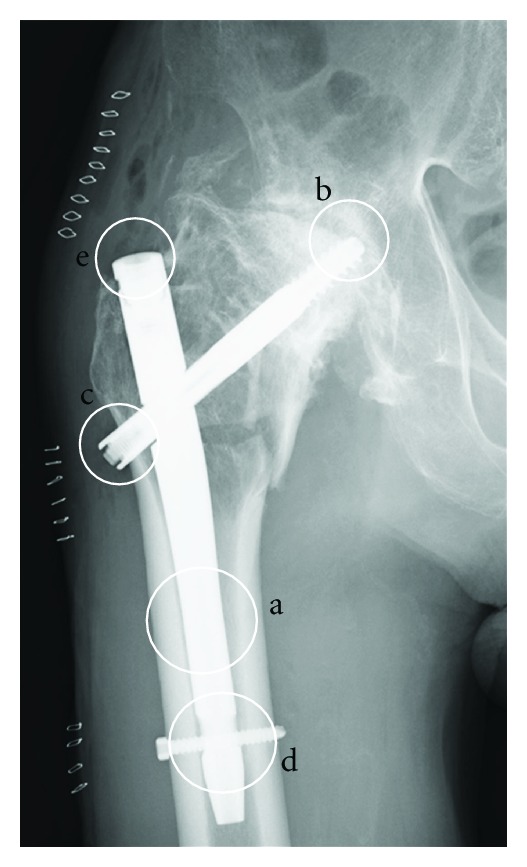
Anteroposterior radiograph after the operation showing the important points of operation for an intertrochanteric fracture of an ankylosed hip, represented with five circles (a–e).
